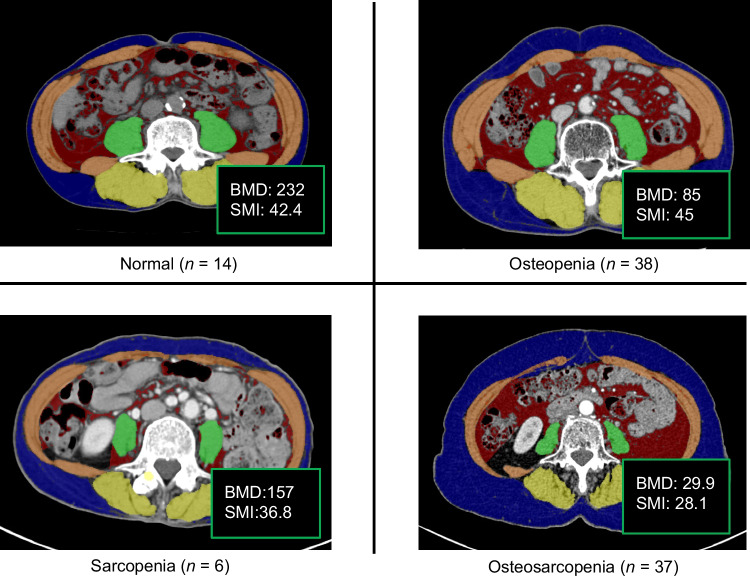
Representative computed tomography images in a normal patient, and in patients with sarcopenia only, osteopenia only, and osteosarcopenia
